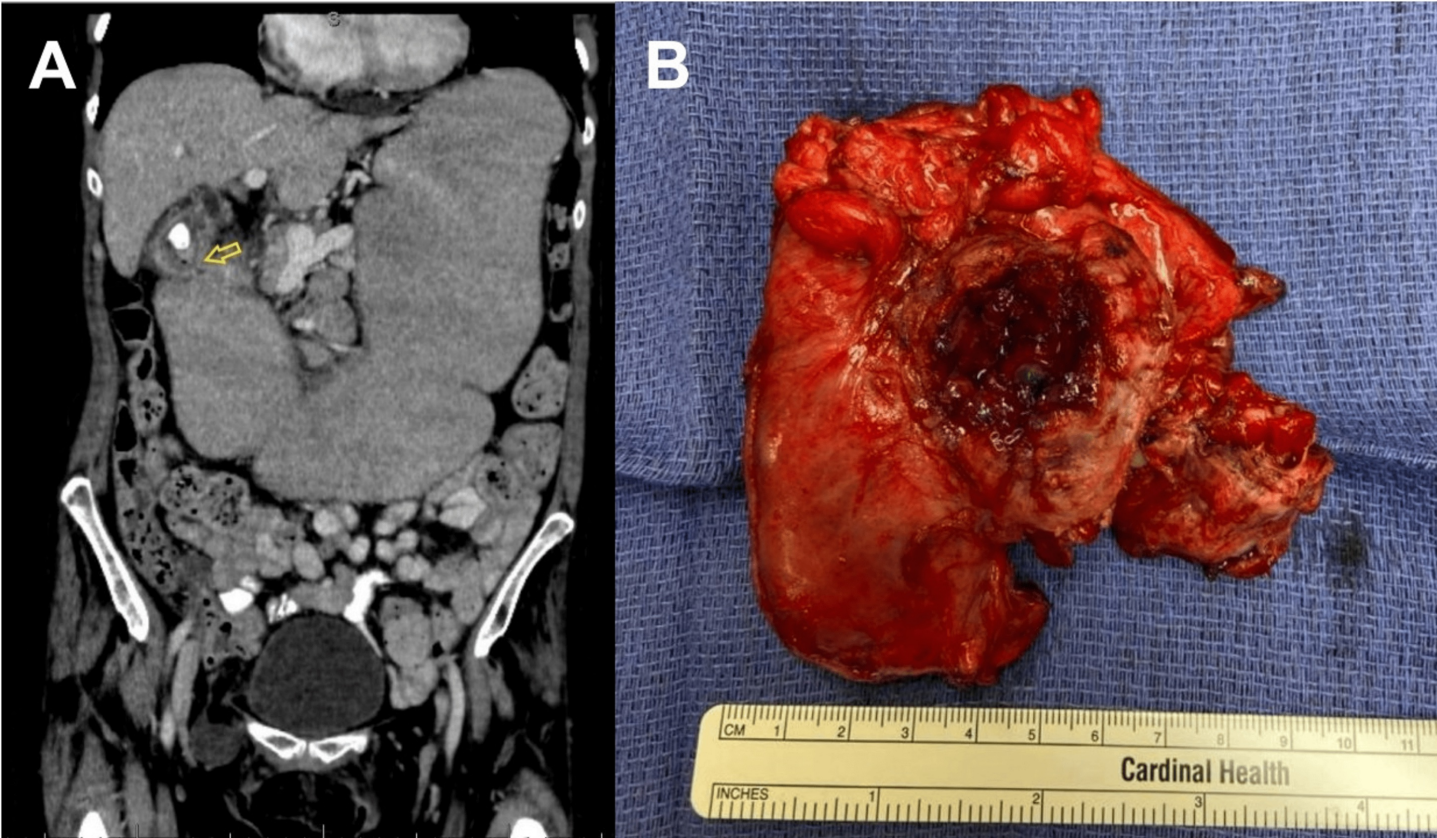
CT of the abdomen and pelvis and intraoperative findings A) Arrow demonstrating retroperitoneal perforation with air and fluid around the duodenum and the cholecystoduodenal fistula on CT of the abdomen and pelvis. B) Intraoperative findings: gallbladder adhered to the duodenum at the site of the stricture and fistula.
